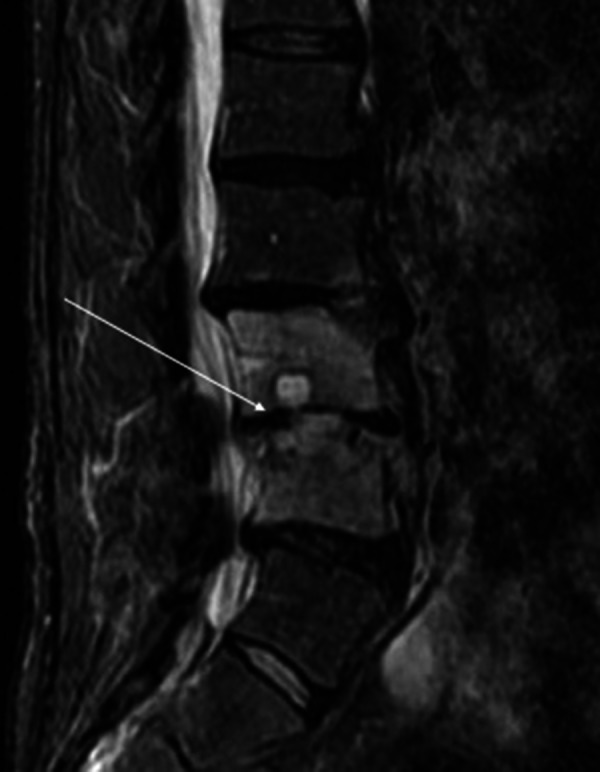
Non-contrast T2 MRI of the spine. There is evidence of osteomyelitis at the L2-L3 and L3-L4 intervertebral levels with abscess in the disc space at the L3-L4 and anterior aspect of the disc at L2-L3.
